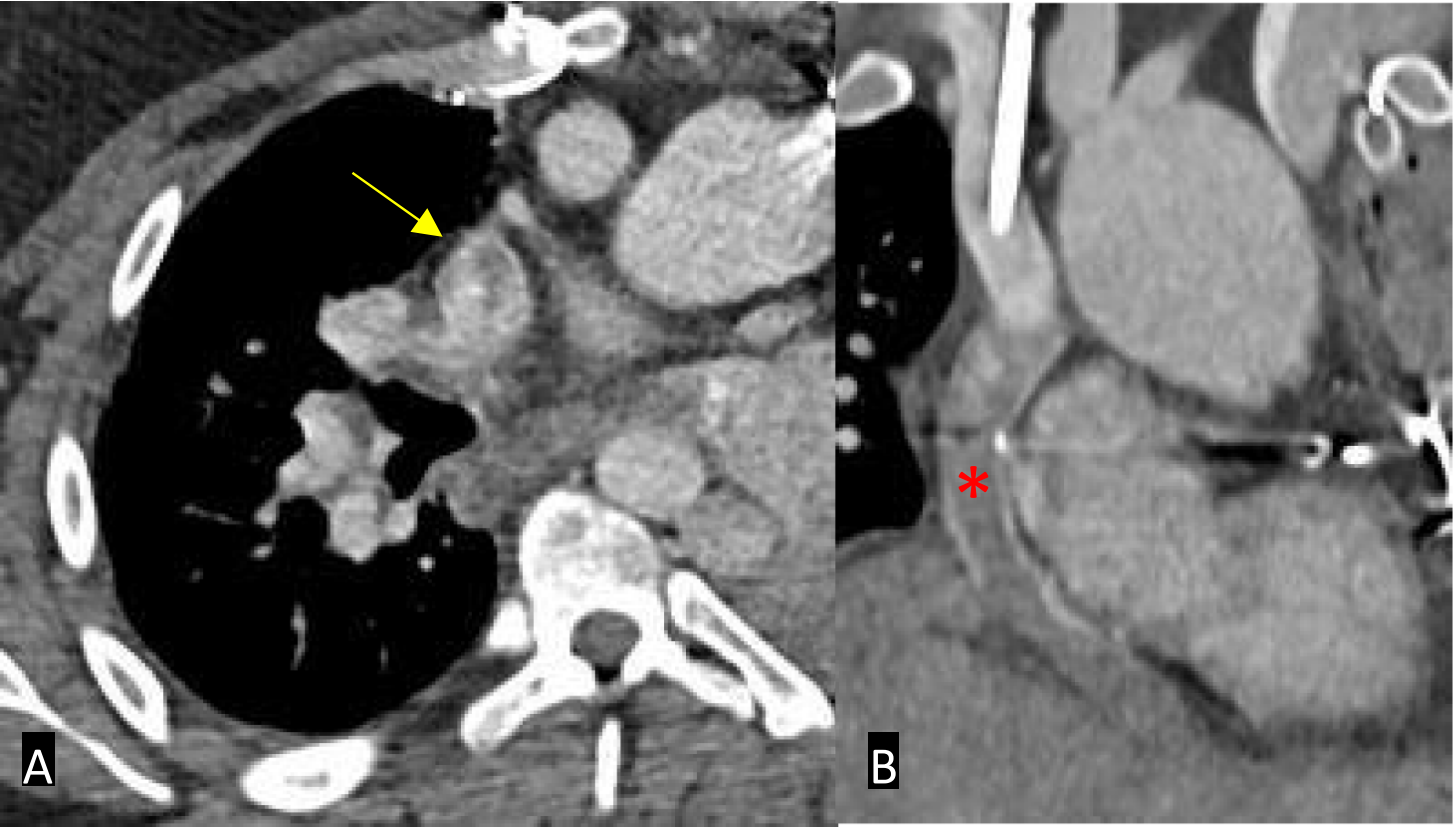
Axial (A) and coronal (B) contrast-enhanced CT images in a patient with an extra-cardiac conduit Fontan circuit. A If the study is protocolled as a standard CT pulmonary angiogram, a pseudo-thromboembolism may be seen – in this case seen in the superior vena cava (yellow arrow). B Note that the conduit also appears poorly opacified (red asterisk)
